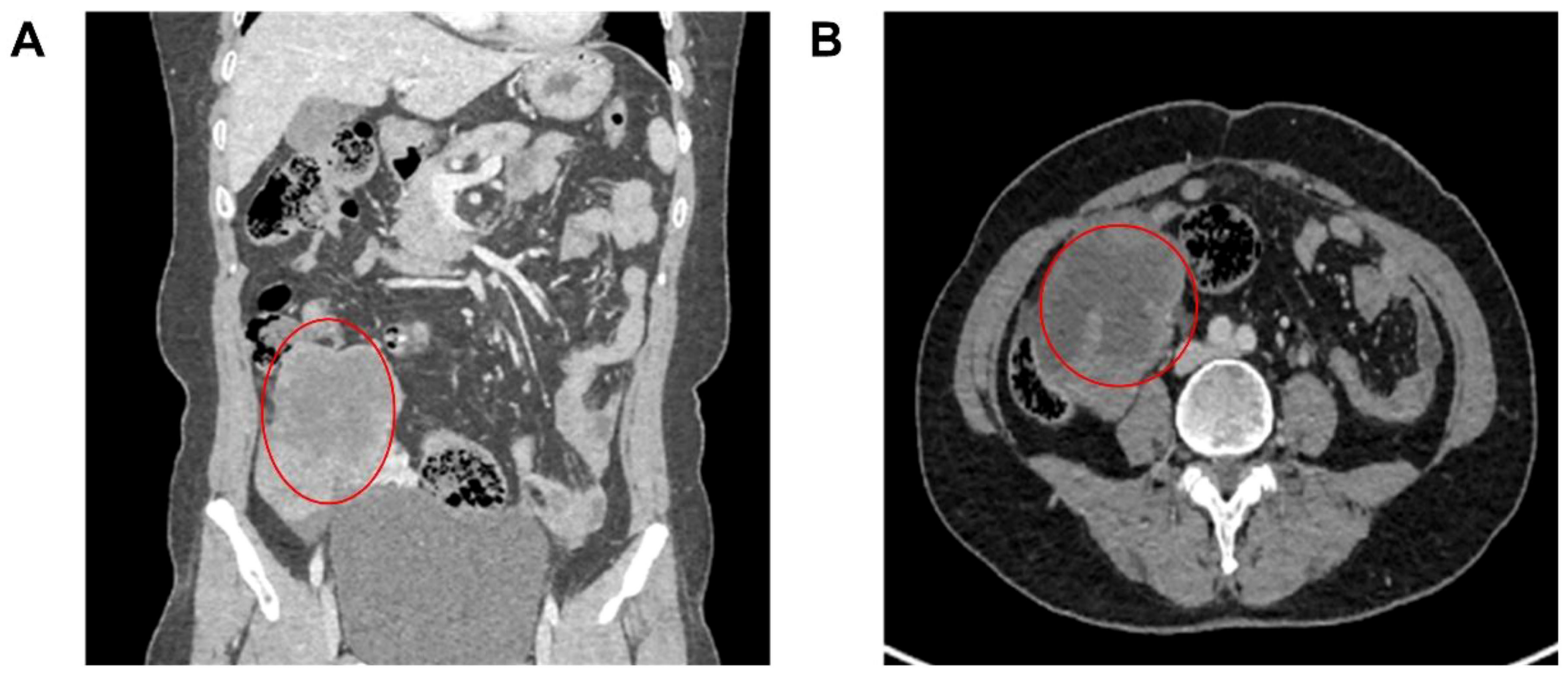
Patient’s CT images. (A) CT showed a solid cystic mass in the right adnexal area; (B) Enhanced CT indicated a suspicious malignant mass in the right adnexal area, and enlarged retroperitoneal lymph nodes. The marked location was a space-occupying lesion in the right adnexal area, with a high possibility of malignancy
